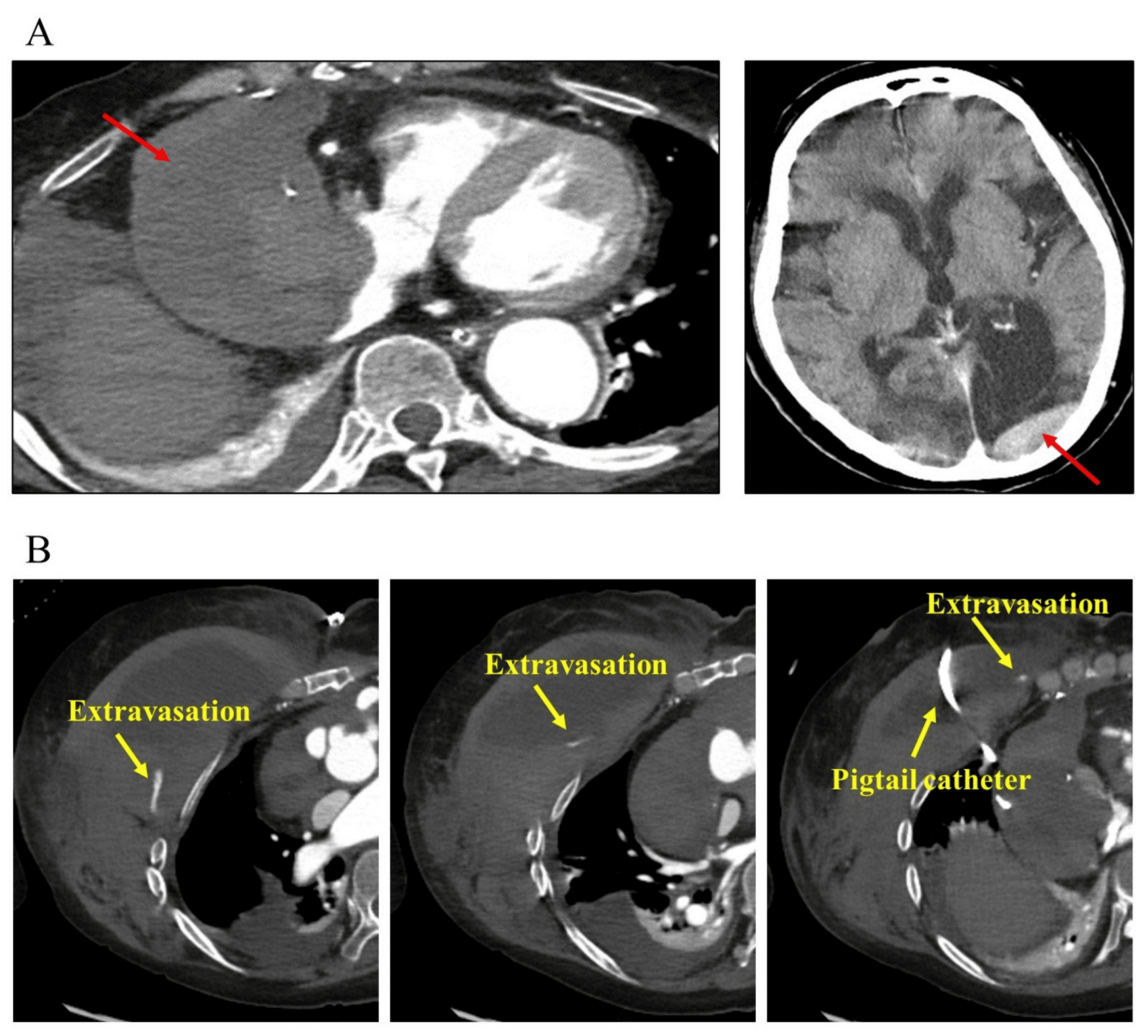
Postoperative computed tomography findings Panel A showed cardiac tamponade due to massive hematoma and subdural hematoma. Panel B showed multiple areas of bleeding within the subcutaneous hematoma
